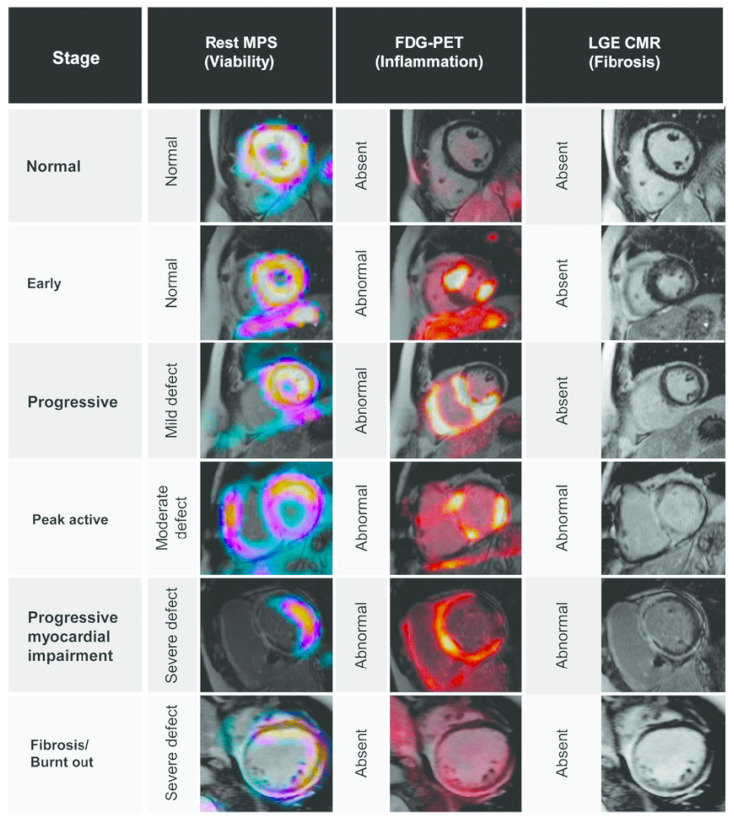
Use of FDG PET for early detection of cardiac sarcoidosis, showing FDG advantages in the ability to detect the activity of inflammation early on, in comparison with resting myocardial perfusion scintigraphy (MPS) and CMR. Figure replicated from [95] under Creative Commons license, provided at https://creativecommons.org/licenses/by-nc/4.0/ (accessed on 1 February 2022). Changes made: Figure legend.
